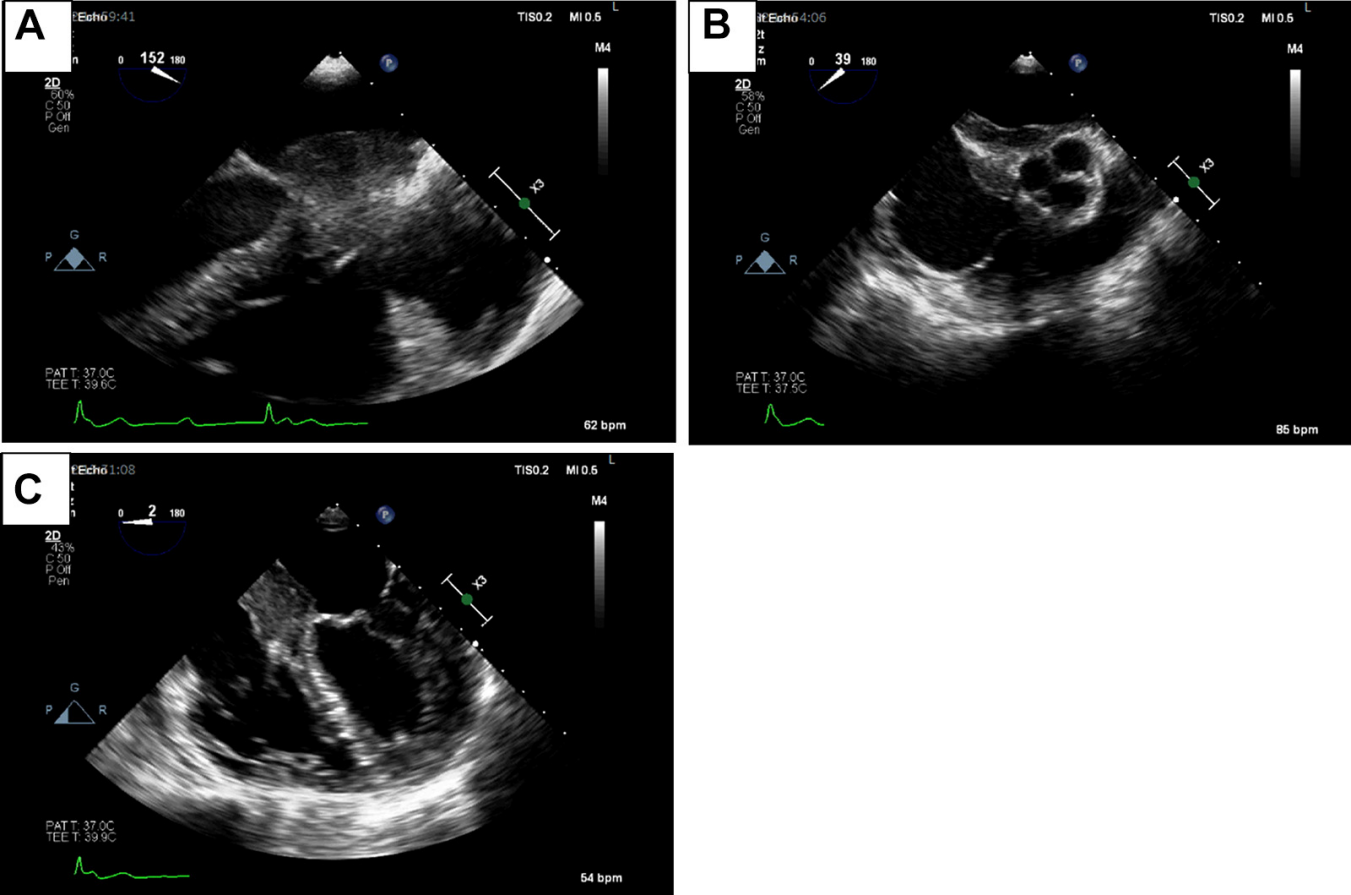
Transesophageal Echocardiogram Transesophageal echo demonstrating the extent of the IAS mass. Abbreviation as in Figure 2.
